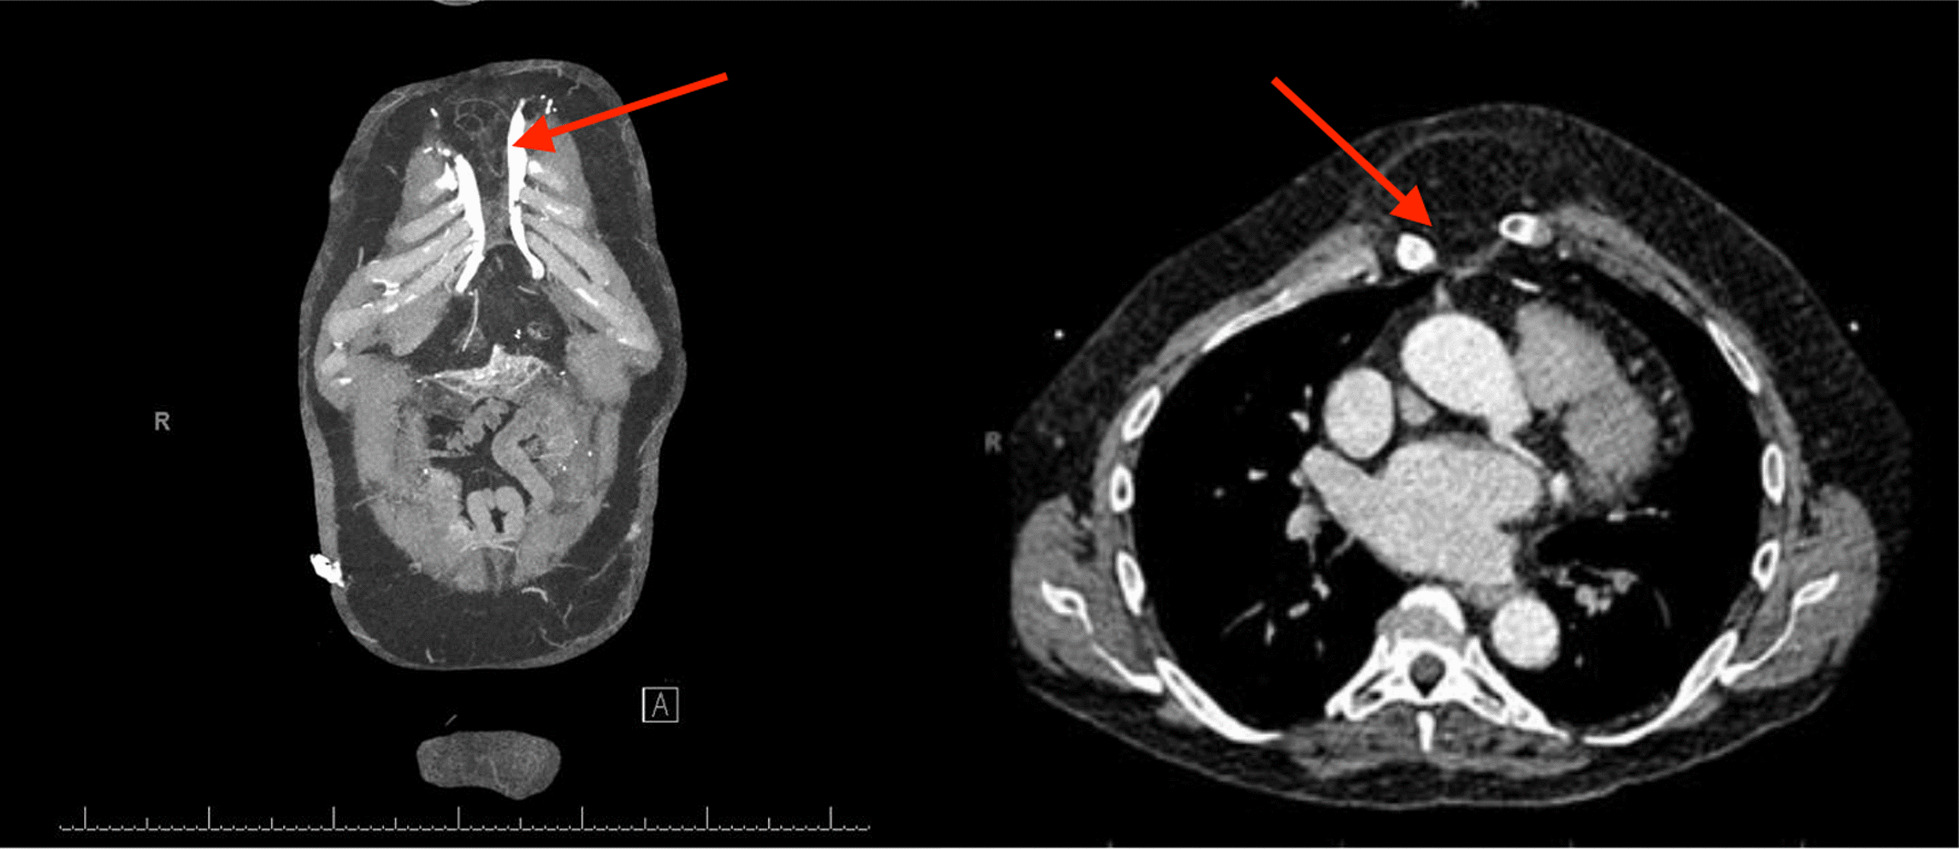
Pre-operative CT scan showing absent sternum
